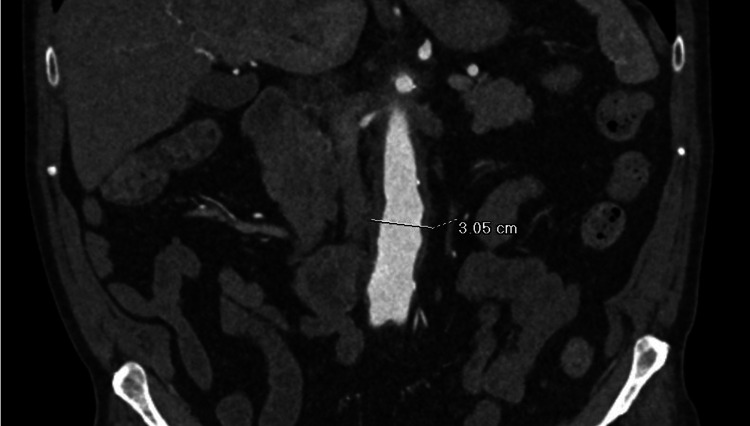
Follow-up CTA five weeks after therapy initiation Coronal image of follow-up CTA five weeks after corticosteroid therapy was initiated showed mild interval improvement in circumferential aortic inflammation. Subsequently, the proximal extent of the aortitis was found to have receded to the start of the abdominal aorta and the thoracic aortic inflammation was resolved CTA: computed tomography angiogram
